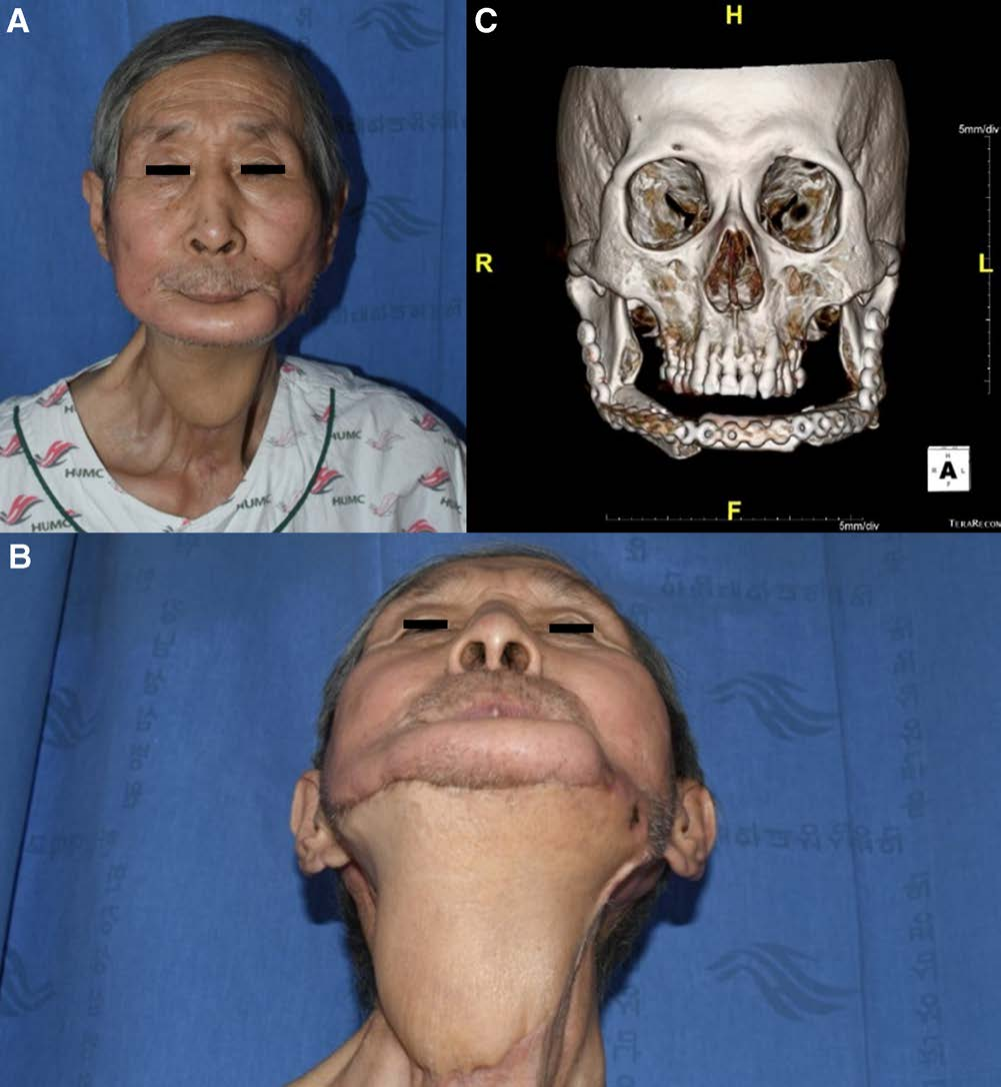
Patient at 20 months postoperatively. Excellent functional outcomes with good speech and swallowing and satisfactory aesthetic outcomes were achieved without flap bulkiness, scar contracture, or cancer recurrence. (A) Front view. (B) Worm’s eye view. (C) Computed tomography. The customized titanium plate was well fixed with osseous segments of the fibula free flap.
